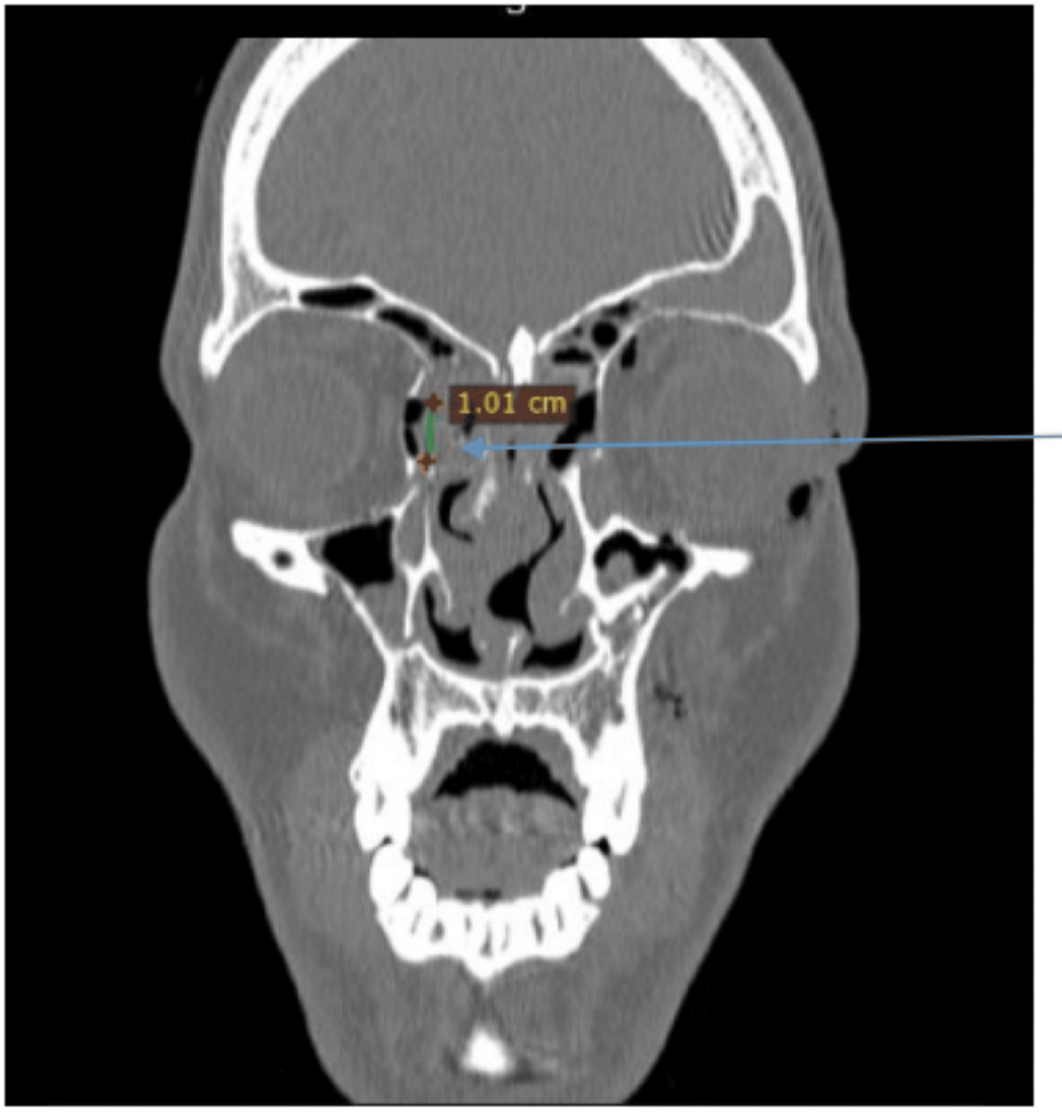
The coronal section of the computed tomography of a fronto-naso-orbito-ethmoid fracture shows the measurement of the disrupted portion of the bony nasolacrimal apparatus. The arrow showcases the measured segment in millimetres and the view of the bony nasolacrimal canal.
